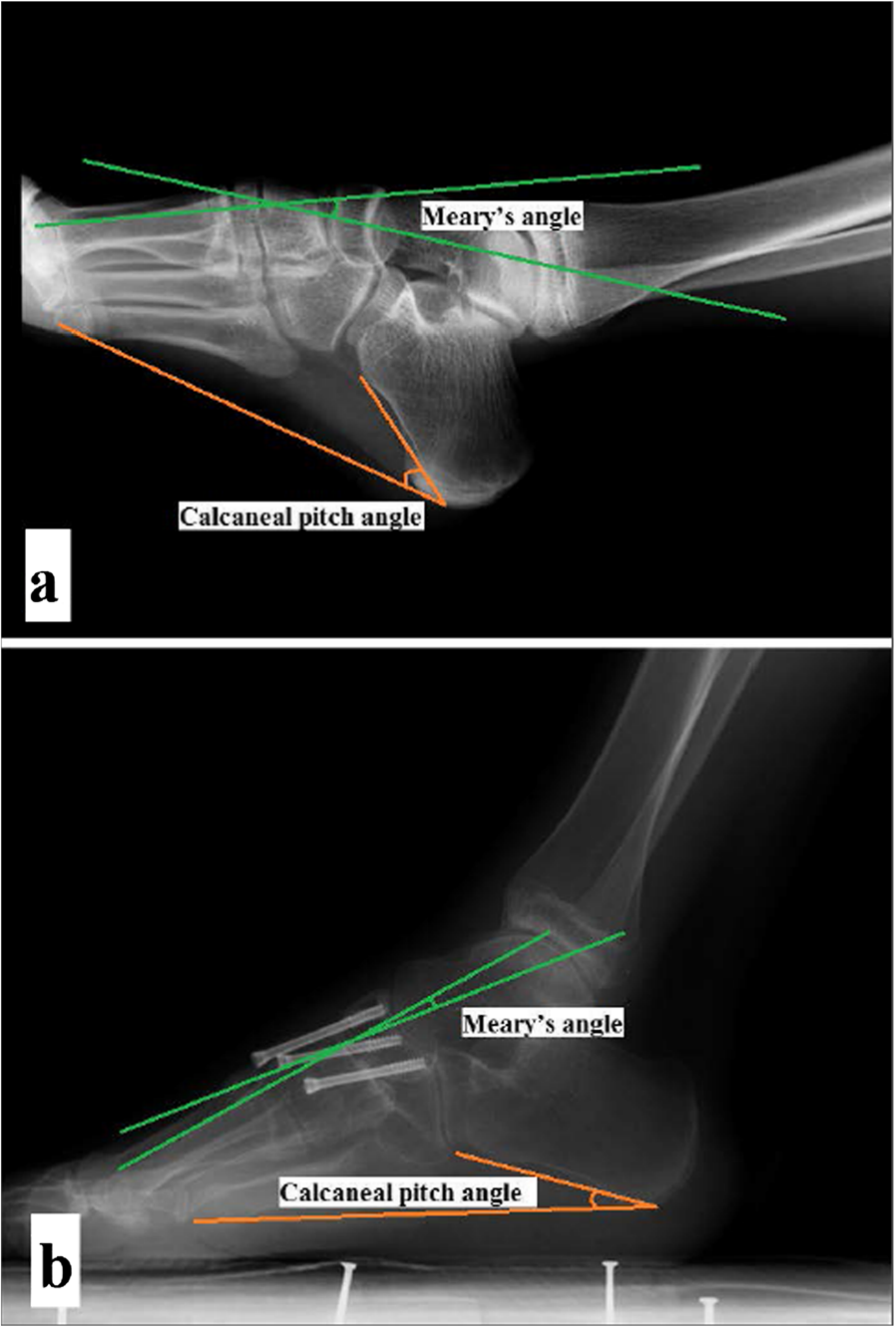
A severe deformity case achieved good correction. A lateral X-ray of a foot (a) shows the preoperative deformity, and (b) a postoperative X-ray of the same patient after midfoot osteotomy and adjacent joint sparing internal fixation is shown.
